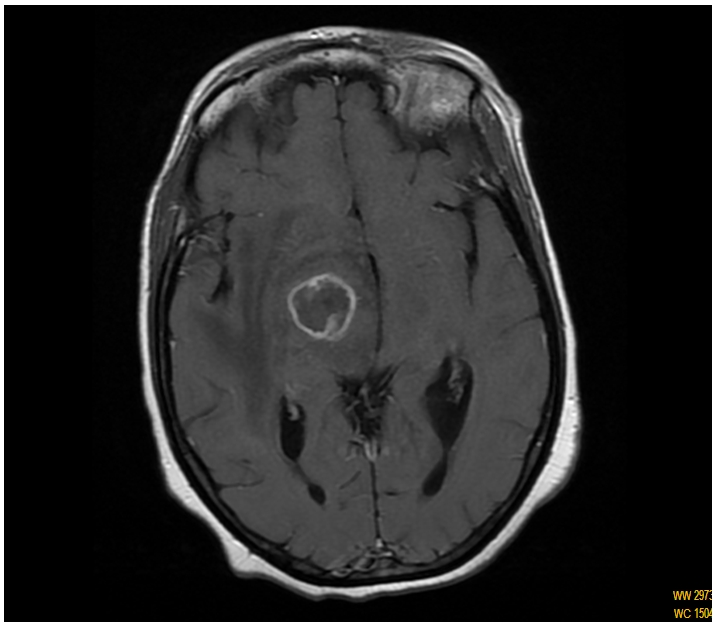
T1-weighted magnetic resonance imaging scan showing ring enhancing of the capsule-thalamic lesion after gadolinium injection in a Moroccan patient with hemichorea-hemiballismus as an initial manifestation of acquired immunodeficiency syndrome and toxoplasma infection
